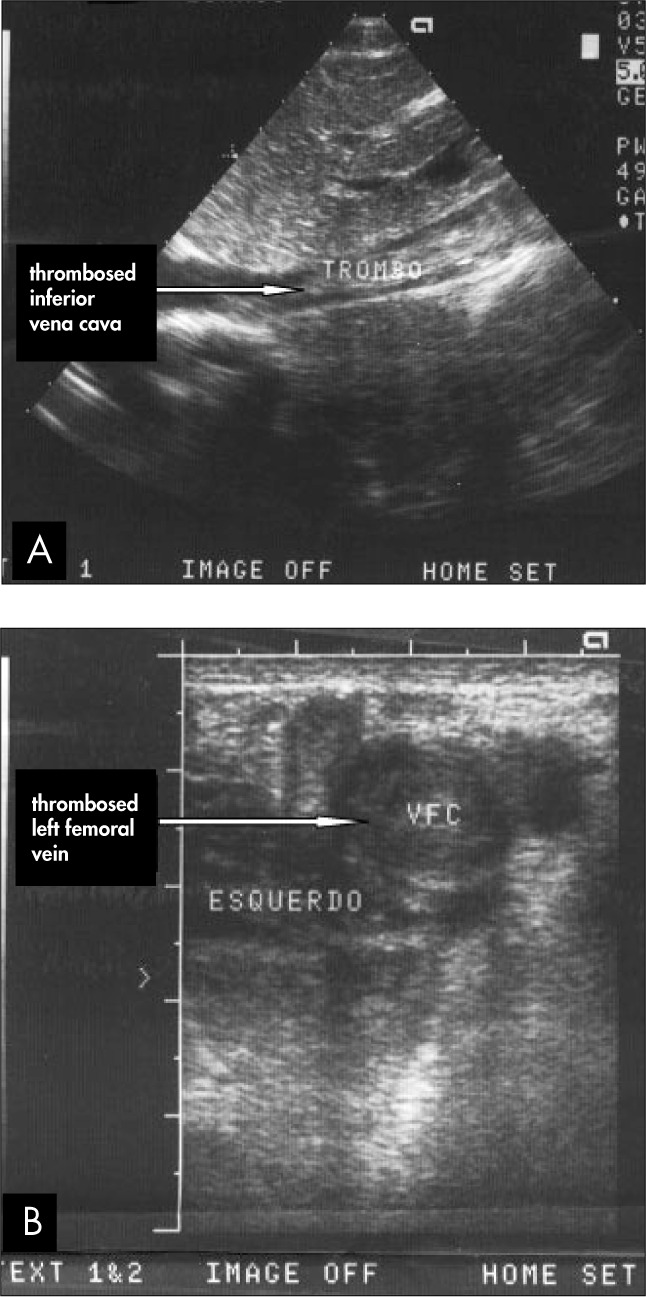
Doppler ultrasound in a 18-yearold cancer patient showing: A) massive thrombosis of the inferior vena cava; B) massive thrombosis of the left femoral vein.
